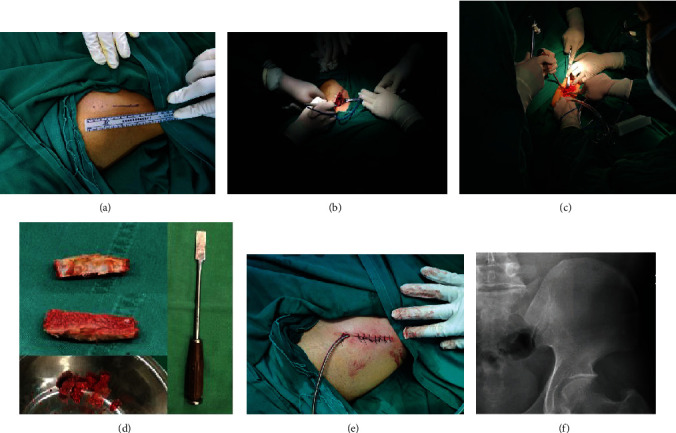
Steps to obtain the structural bicortical autologous iliac crest bone. (a) Locate the bony markers of the iliac crest and predict the surgical incision; (b) soft tissues were dissected along the lateral lip of the iliac crest with an electrome; (c) a special sharp narrow chisel was used to harvest a bicortical bone segment; (d) structural bicortical autologous iliac crest bone segment, cancellous bone, and bone harvest tool; (e) surgical incision at the bone-graft donor site; (f) postoperative radiograph of the bone-graft donor site.
